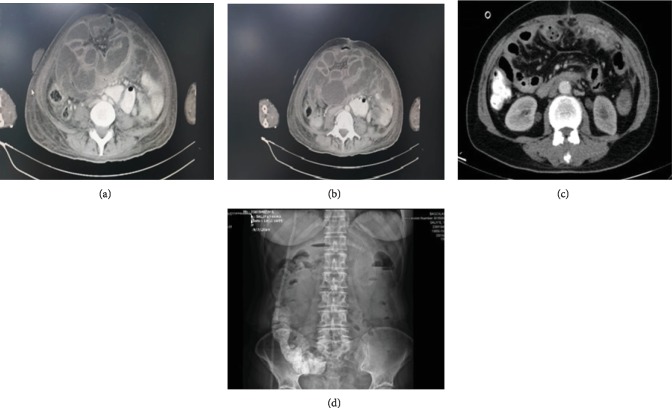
Computed tomography and plain abdominal X-ray of the patients with complete type 3 ACS. Case 3: (a) and (b). Case 4: (c) and (d).
